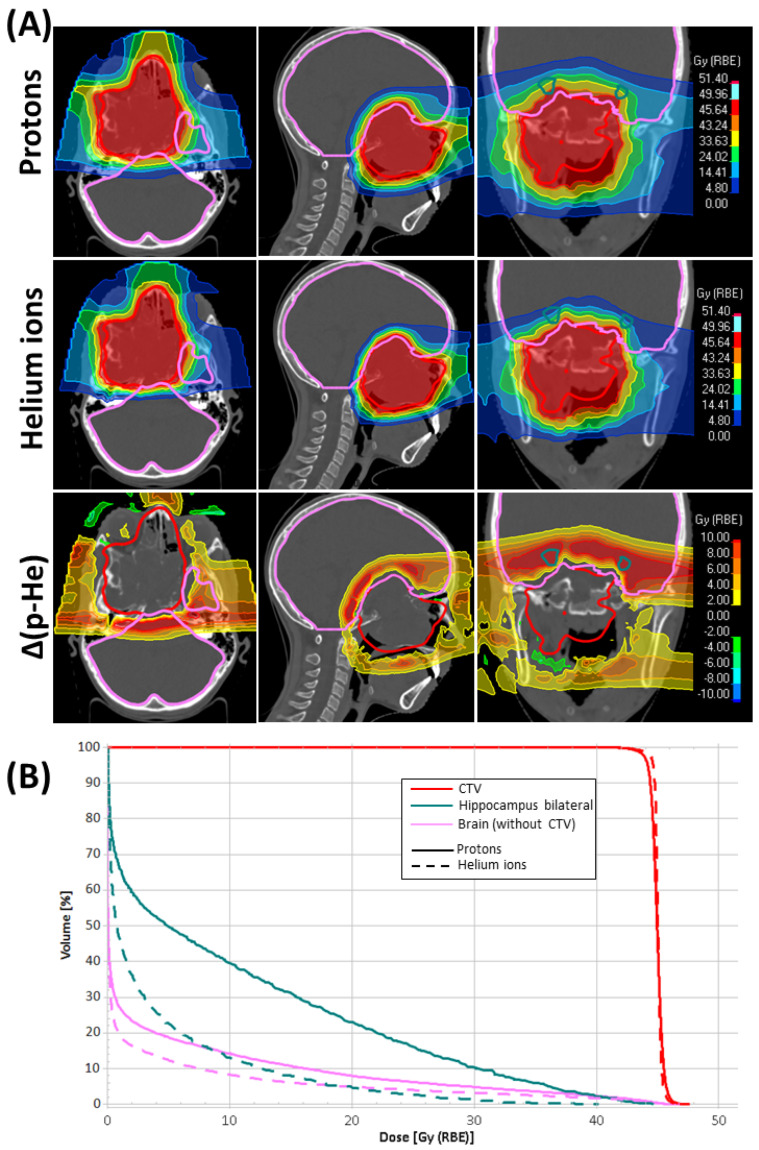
(A) Dose distribution on the axial, sagittal and coronal planning CT slices for an exemplary patient with protons and helium ions and the dose difference between the two modalities (Δ(p-He)). (B) Dose–volume histograms (DVH) for selected volumes for both modalities. The clinical target volume (CTV) is displayed together with bilateral hippocampus and brain (without the CTV) on the dose maps and DVH. DVH from protons and helium ions plans are represented with solid and dashed lines respectively.
